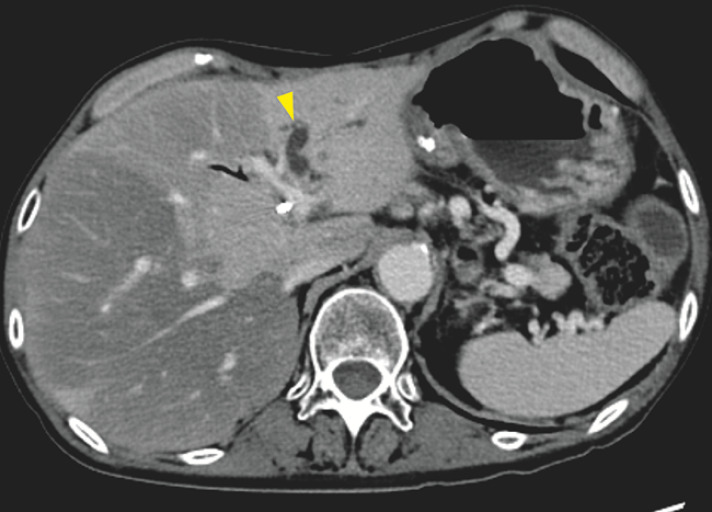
Contrast-enhanced computed tomography image of the dilated left hepatic bile duct (yellow arrowhead), which was obstructed by metastasis of pancreatic cancer on the biliary hilum.
